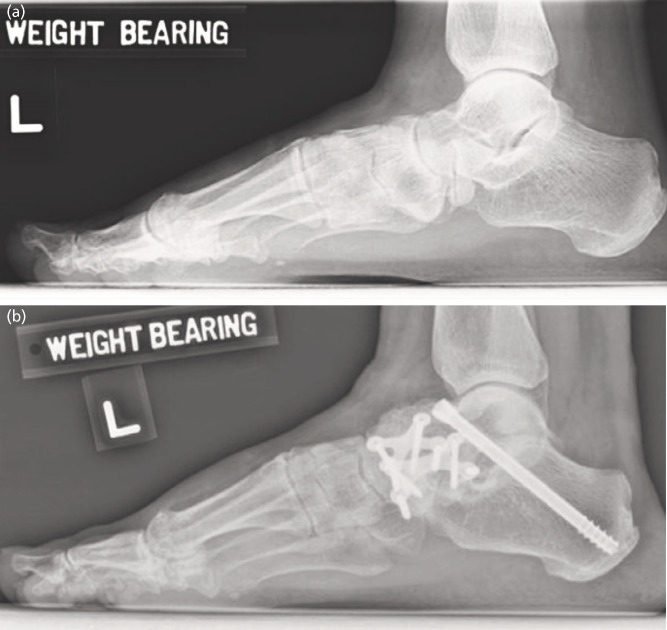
Weight bearing radiographs (a) pre-surgery and (b) six months post-surgery.
